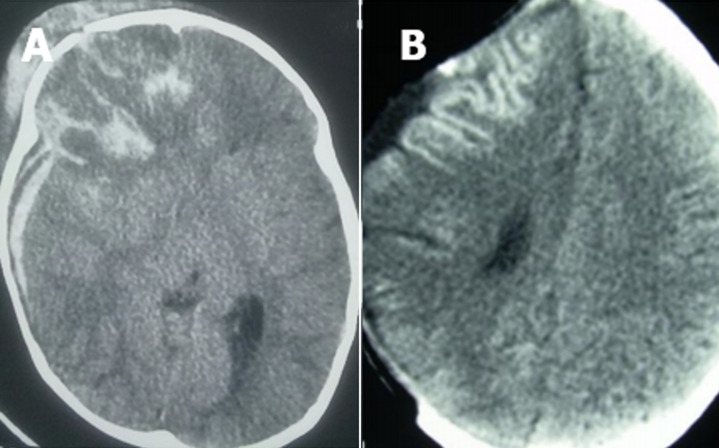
A) pre-operative axial section brain CT scan of a right oedemato-hemorrhagic frontal contusion associated with temporal (HSDA); B) decompressive post-craniectomy brain CT scan of right oedemato-hemorrhagic frontal contusion associated with temporal HSDA in the same patient
